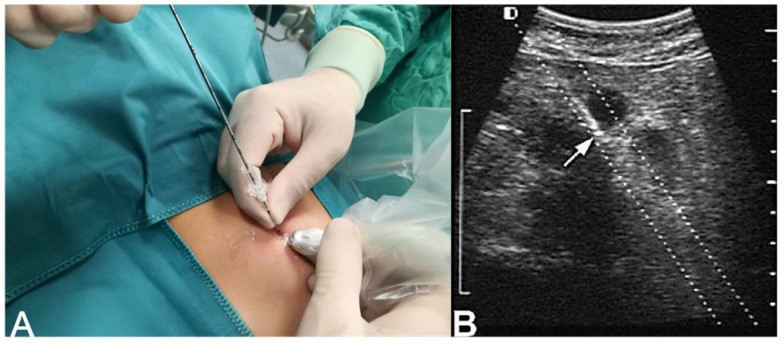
(A). Ultrasound scan of a large adrenal tumor. (B). Ultrasound-guided core needle biopsy (the arrow shows the tip of the needle within the adrenal gland).
